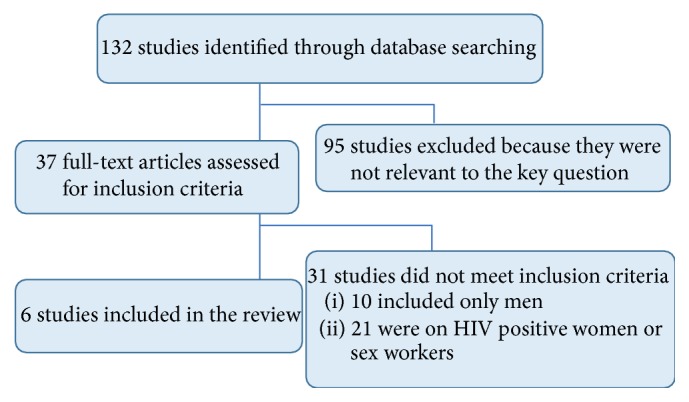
Table 1 tab1: Characteristics of the studies addressing *Chlamydia trachomatis* (CT) prevalence among childbearing age women in India.
